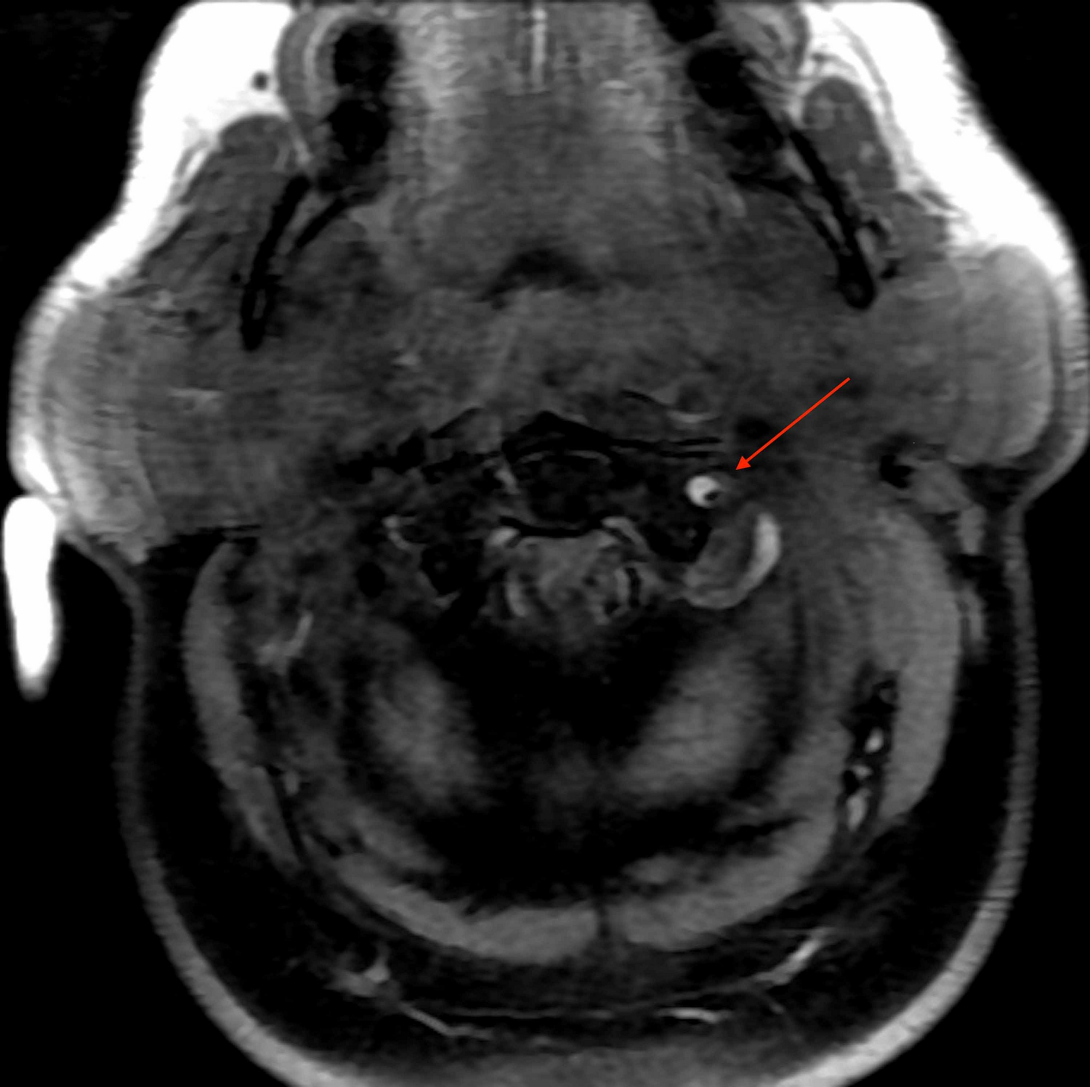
Axial T1 MRI at the C1-C2 level demonstrates T1 hyperintense crescent-shaped false lumen with T1 hypointense narrowed true lumen.
